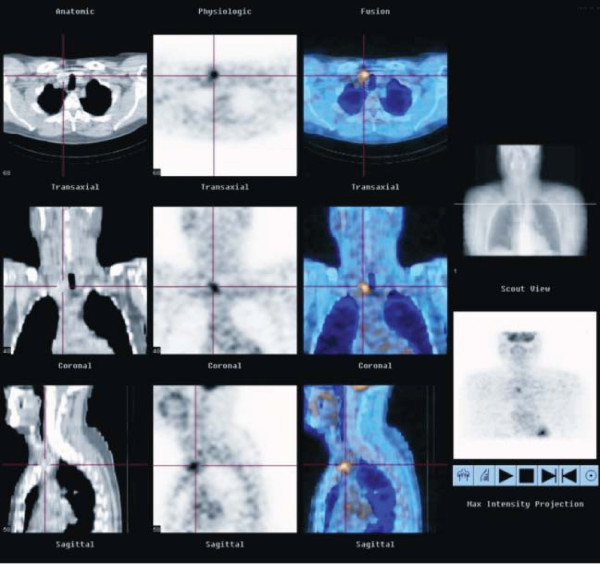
^18^F-FDG PET/CT scan demonstrating focal intense ^18^F-FDG uptake in the nodule in the inferior pole of the right lobe of thyroid.
